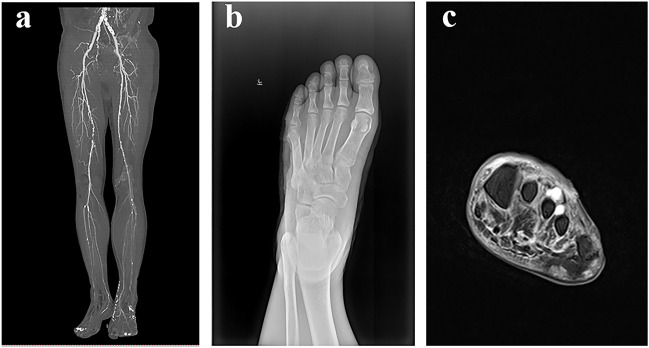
Aids to the examination. (A): Below-knee CTA showed that lower limbs with arteriosclerosis and lumen had various degrees of stenosis. (B) Foot skew radiographs showed that the soft tissue density of the lateral left foot was non-uniform and locally absent. (C) Foot MRI showed that there was no obvious abnormality in the left bone marrow, plantar and dorsal regions of the foot muscle gap had a flocculently visible signal on T2, and plantar fascia thickening.
